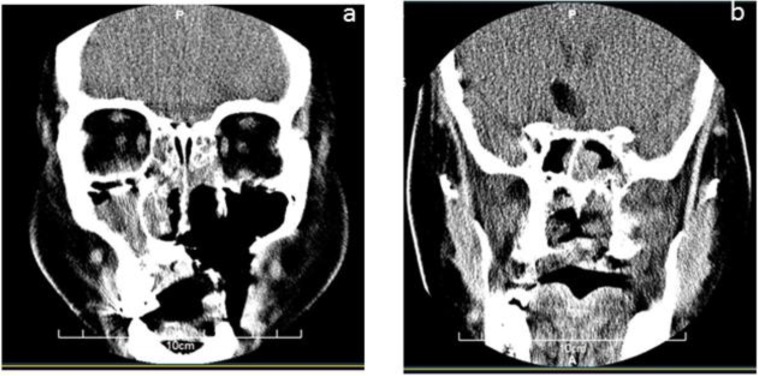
a: there is evidence of bone destruction and surgical resection in right maxillary sinus and right side palate; b: CT scan of face and paranasal sinuses, there is evidence of air fluid level in both maxillary sinus compatible with acute sinusitis. Mucosal thickening is also seen in ethmoidal and sphenoid sinuses
